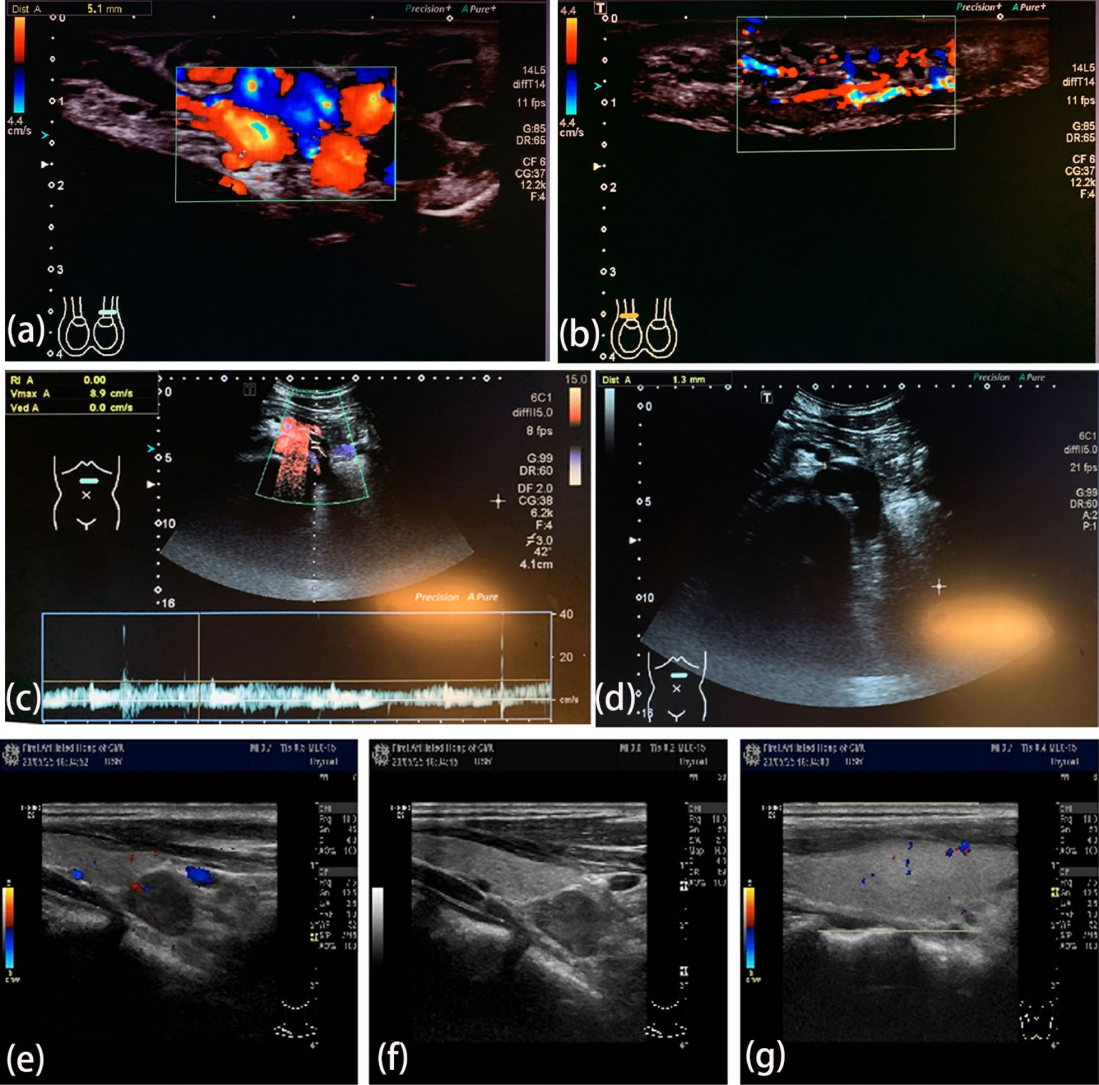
Ultrasonography revealed the left varicocele, nutcracker phenomenon, and right parathyroid nodule. a The left varicocele. b The right normal spermatic vein. c, d The left renal vein was compressed across the abdominal aorta. e, f, g Neck ultrasonography showed one hypoechoic nodule under the right lobe of the thyroid
